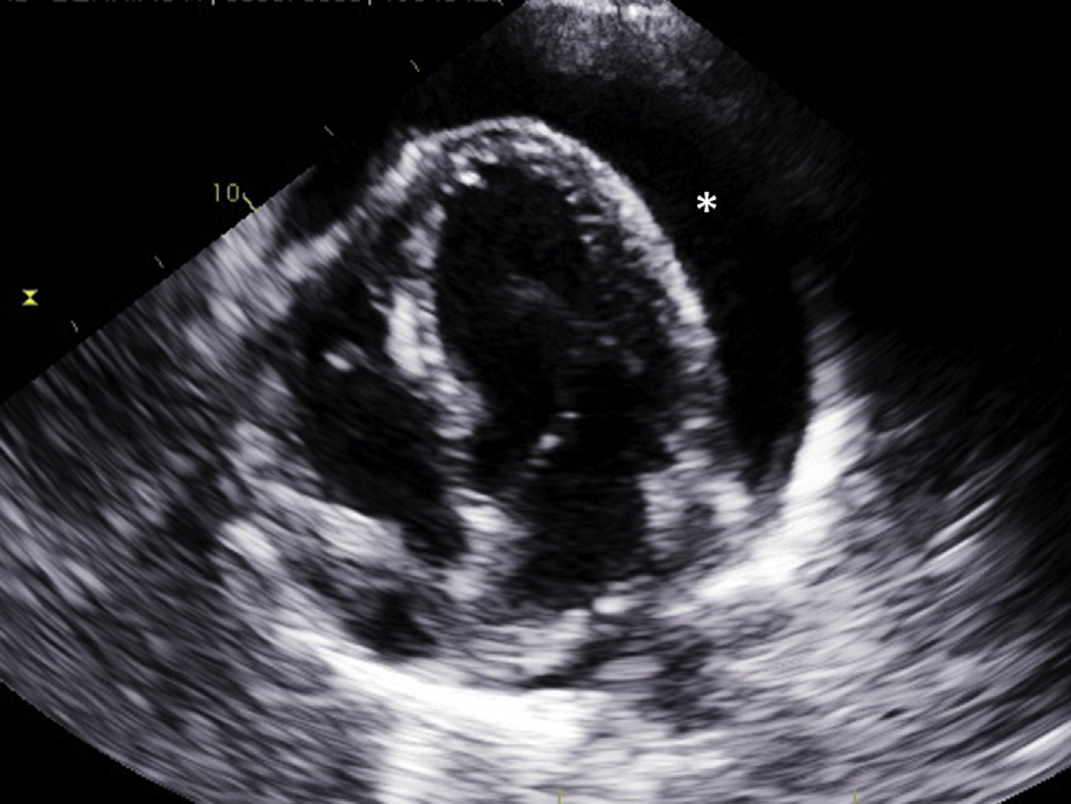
Echocardiography Transthoracic echocardiogram showing pericardial tamponade (asterisk).
